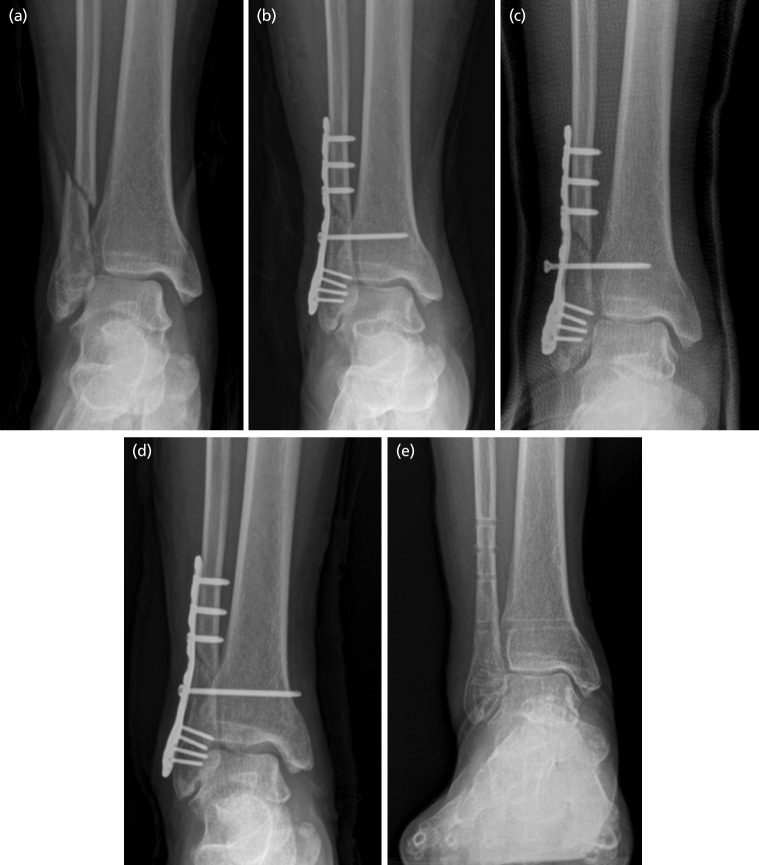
Complication of screw fixation. (a) Pre-operative anteroposterior radiograph of right ankle showing supination external rotation-type ankle fracture. (b) The syndesmosis was fixed with a cortical screw. (c) At four weeks post-operatively, the syndesmosis reduction was loss due to screw cut out. (d) The syndesmosis was re-fixed with a cortical screw. (e) At the one-year follow-up, all implants were removed; radiograph showing complete bone union and well-maintained ankle mortise.
